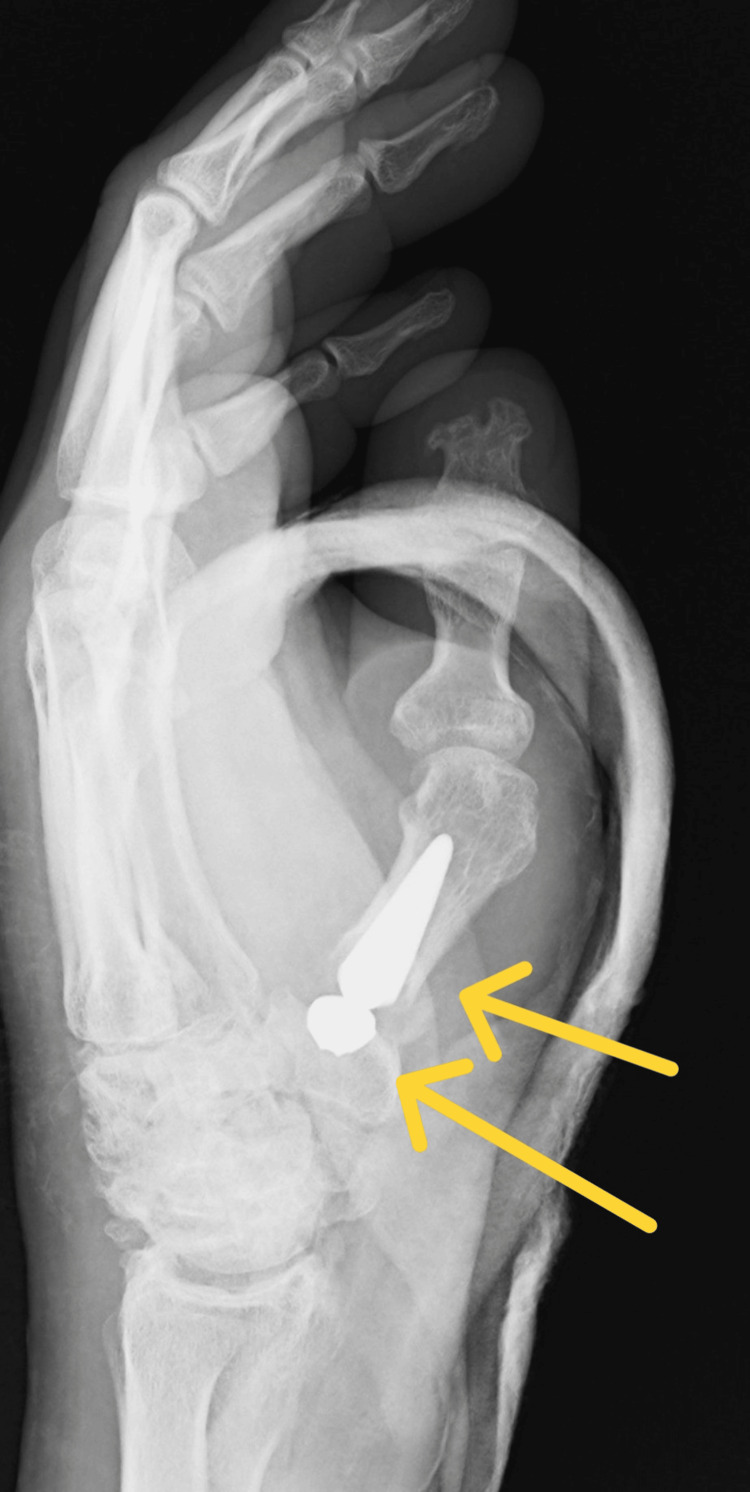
Control X-ray in lateral projection obtained after surgery
